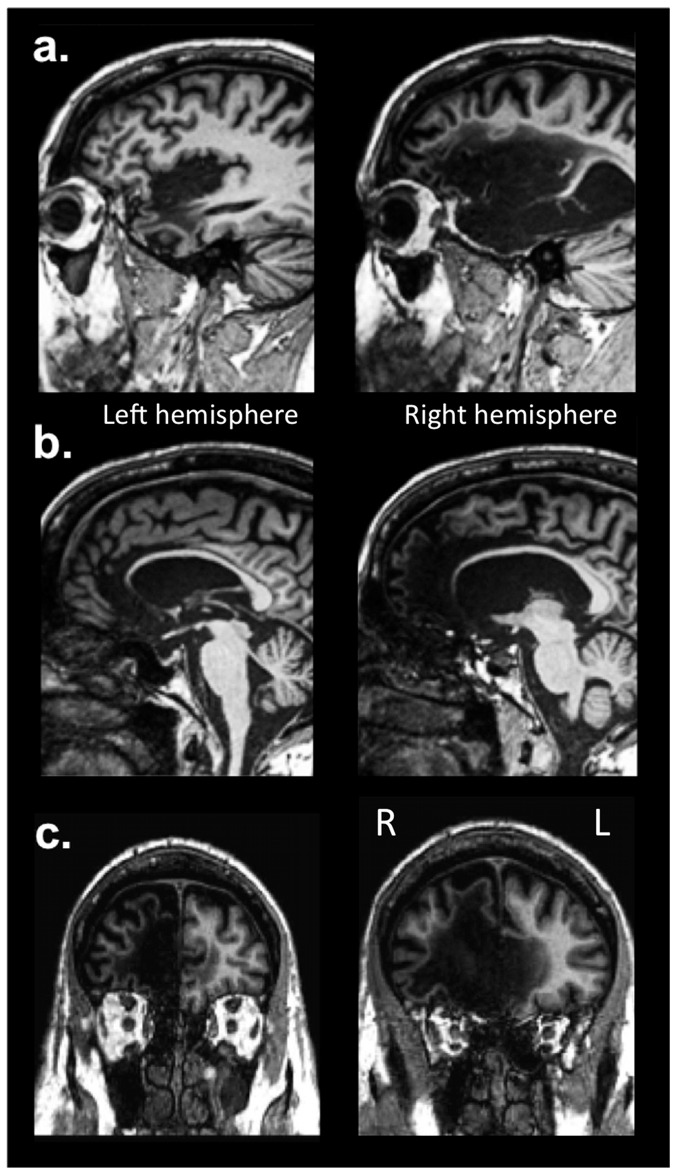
T1-weighted MRI scans of R's lesion. (a) Sagittal images highlighting damage to the insular cortex, (b) sagittal images highlighting damage to the ACC, and (c) coronal images highlighting damage to the mPFC. All three structures are extensively damaged, bilaterally, in R's brain.
